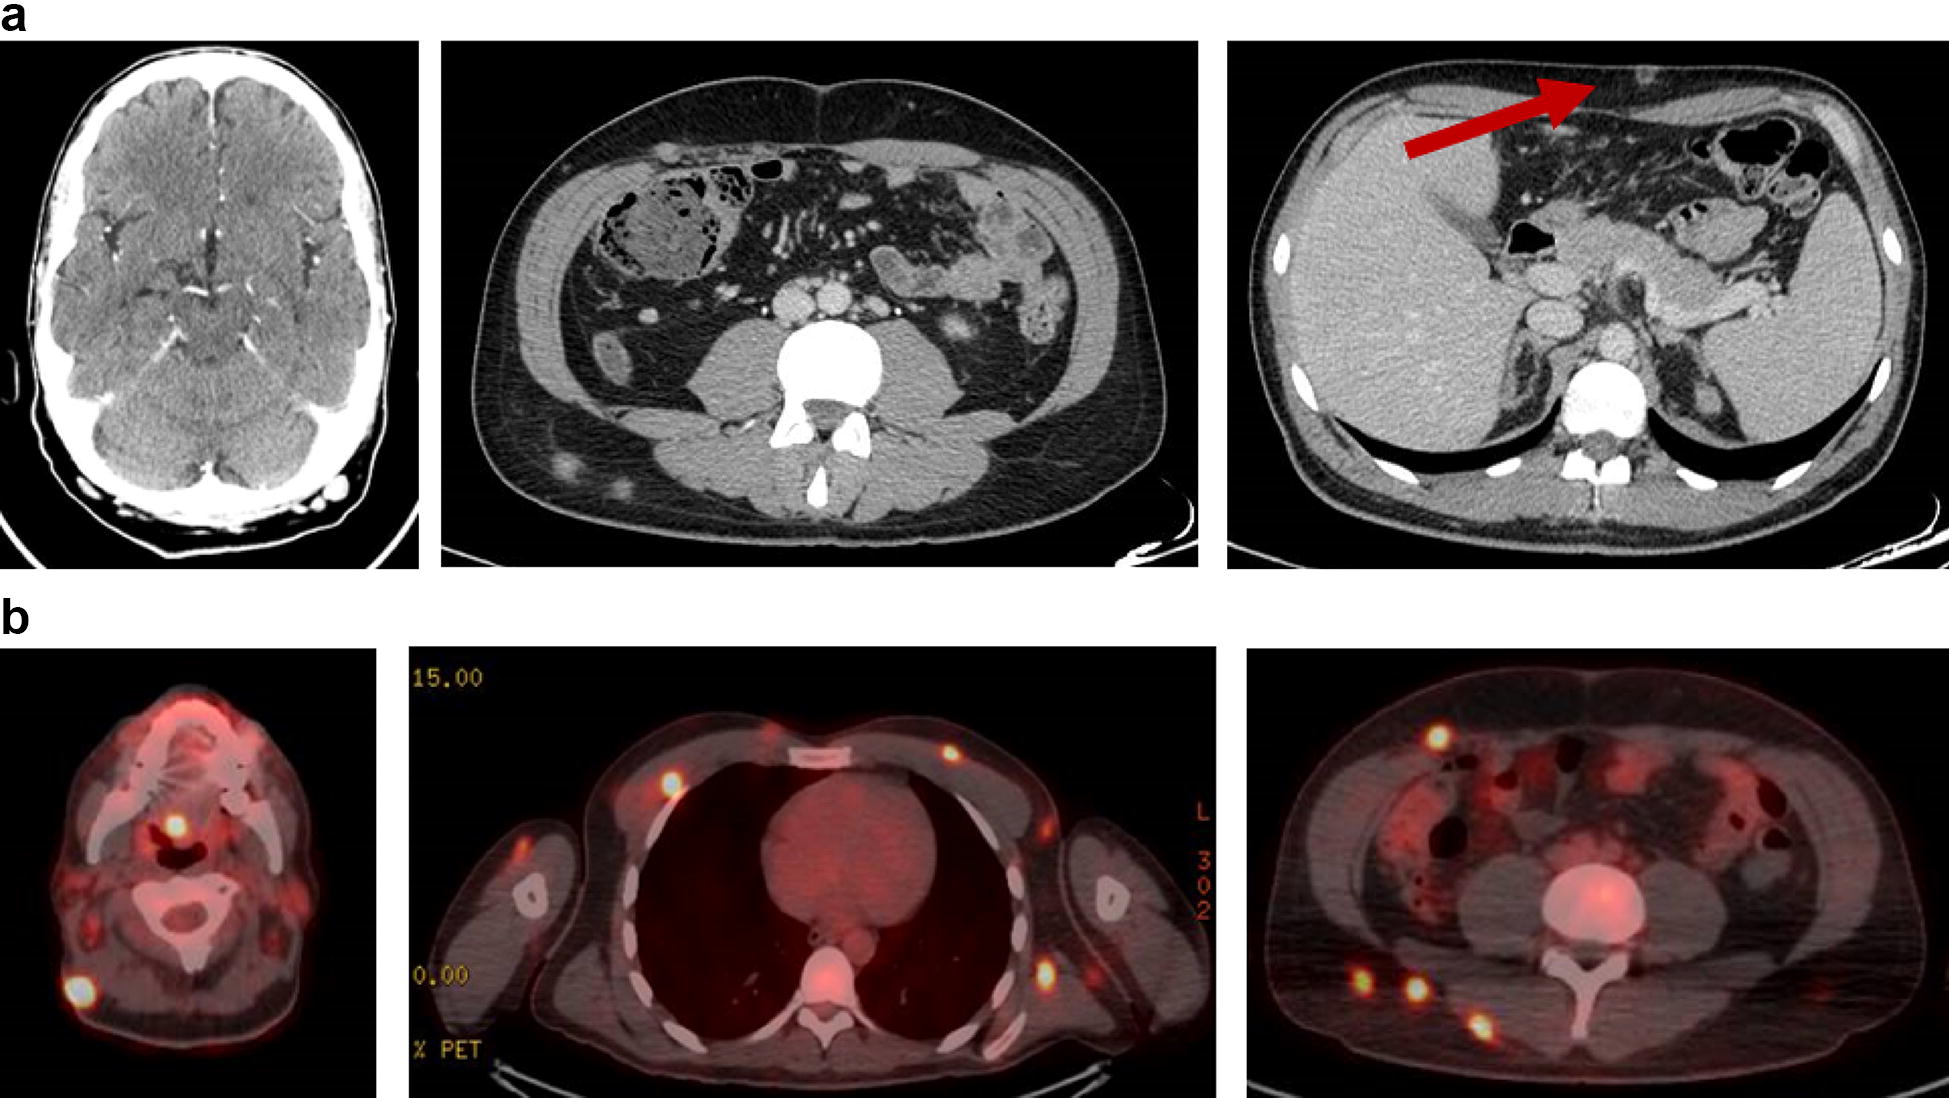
Radiologic exams. a Total body CT scan. Several subcutaneous solid masses with irregular shape and contrast enhancement. (arrow on the excised lesion). b PET-CT total body scan. Ubiquitous hyperfixating lesions, distributed both over and under the diaphragm
